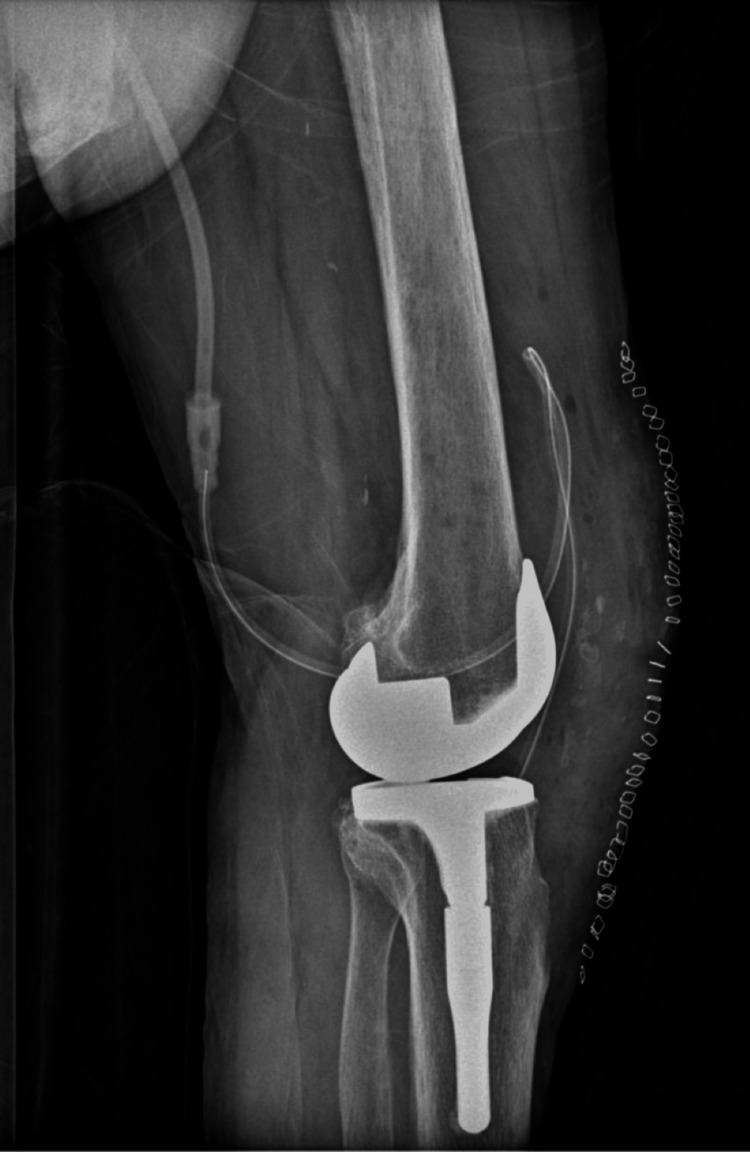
Postoperative Lateral X-ray of the Left Knee
